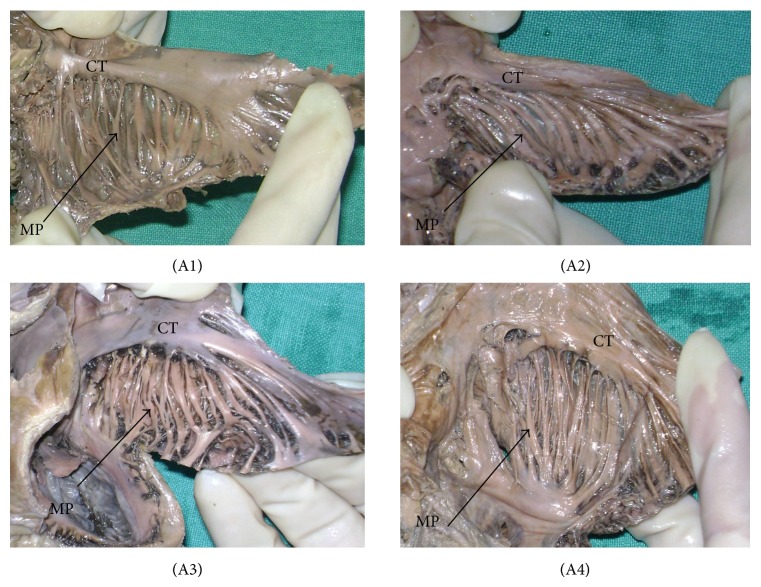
The patterns of musculi pectinati (MP). The black arrow indicates MP. CT = crista terminalis. (A1) type 1, (A2) type 2, (A3) type 3, (A4) type 4.
